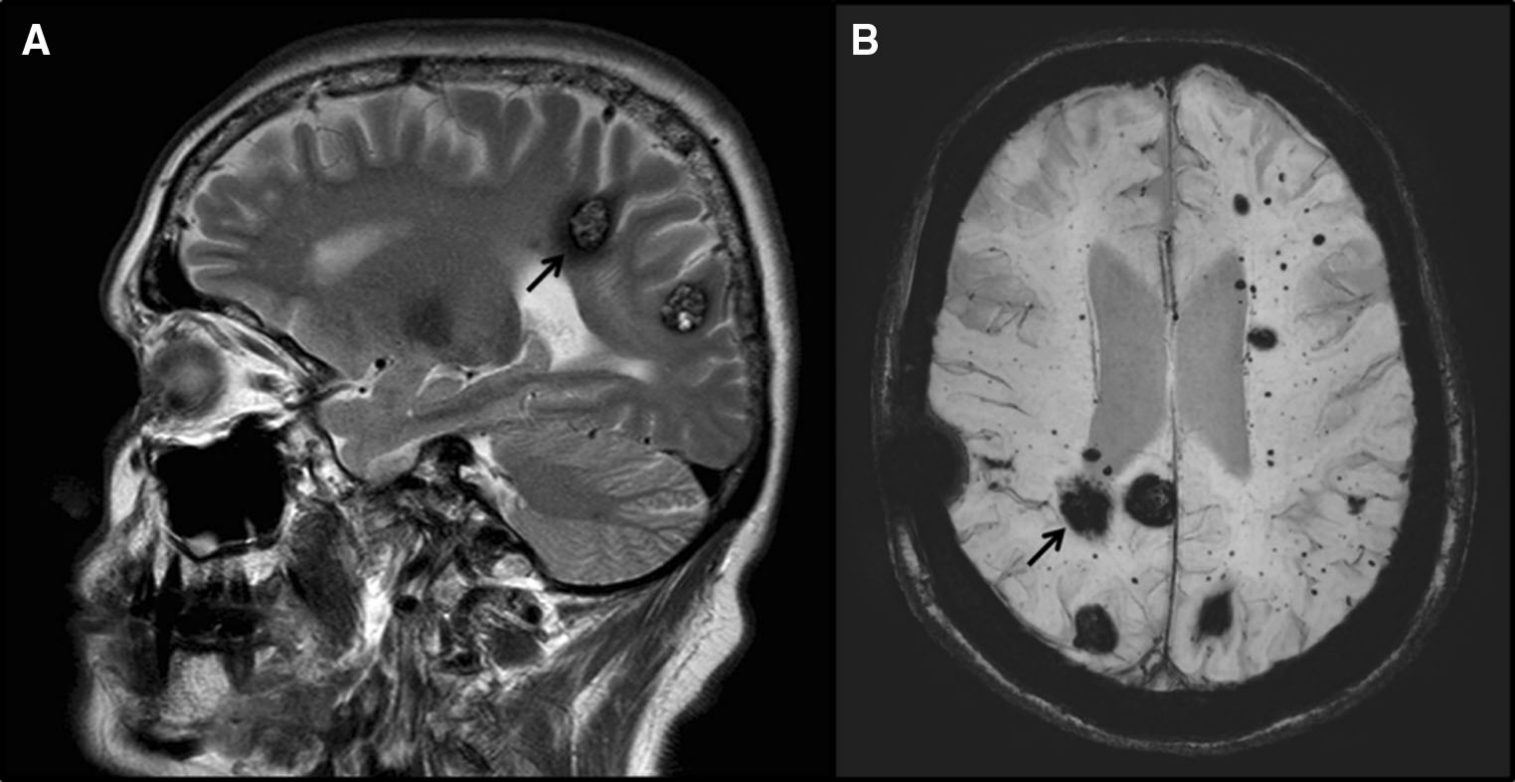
Cerebral magnetic resonance imaging scan of proband showing multiple haemangiomas and cavernous malformations
